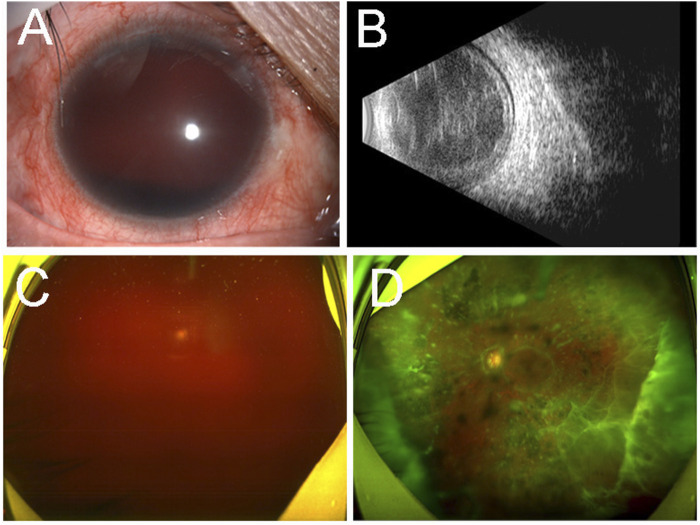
Ocular imaging findings of the both eyes of the proband (III-13) four years later. Slit-lamp examination (A) revealed hyphema of the right eye. B-mode ultrasonography (B) showed mass echoes in the vitreous cavity. Ultra-wide-field scanning laser ophthalmoscopy revealed vitreous hemorrhage in the right eye (C) and multiple flocculent degenerations in the vitreous of the left eye (D).
